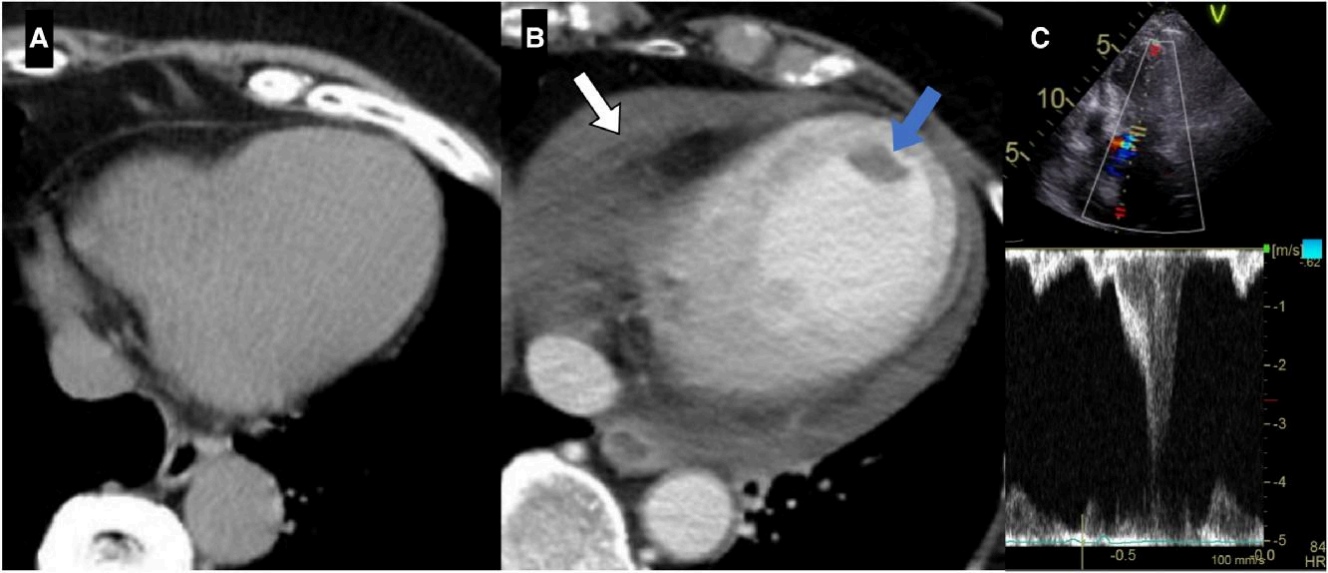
(*A*) Plain computed tomography on admission shows no pericardial effusion. (*B*) Contrast-enhanced computed tomography on day 6 reveals a left ventricular apical thrombus (blue arrow) and moderate pericardial effusion suspected to be haemorrhagic (white arrow). (*C*) Transthoracic echocardiography on day 6 reveals left ventricular outflow tract obstruction with a peak velocity of 4.0 m/s.
